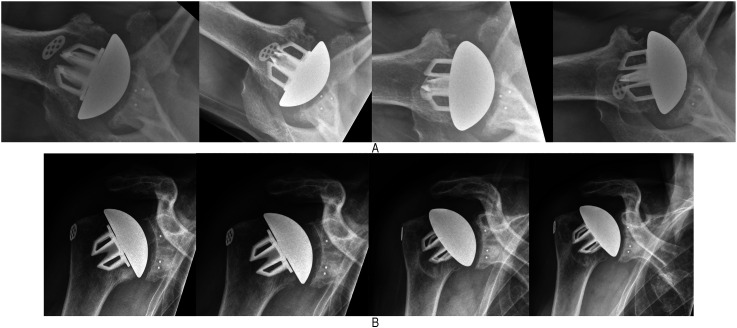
(A) sequential axillary radiographs (L to R: 6 weeks, 6 months, 12
